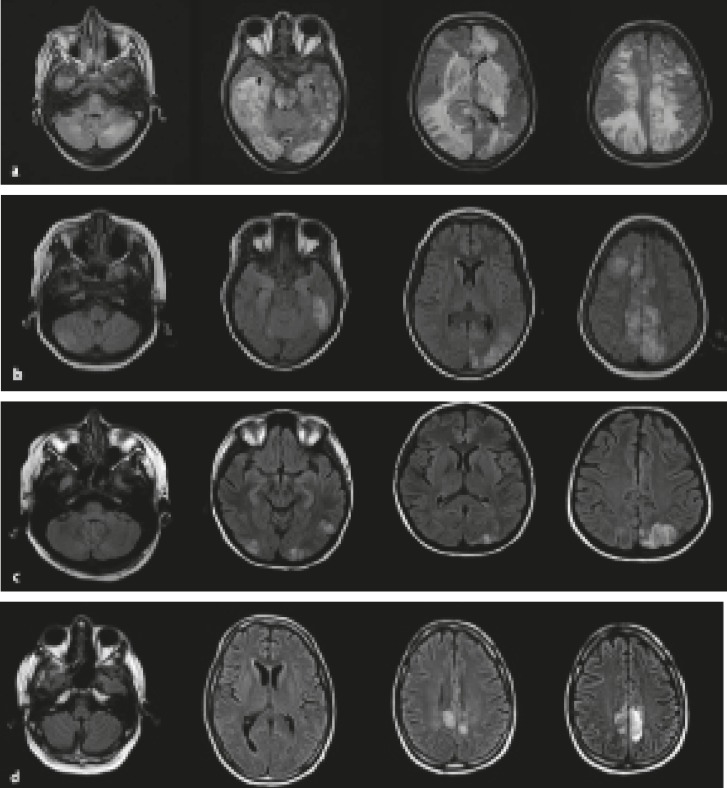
Four main MRI patterns of PRES
